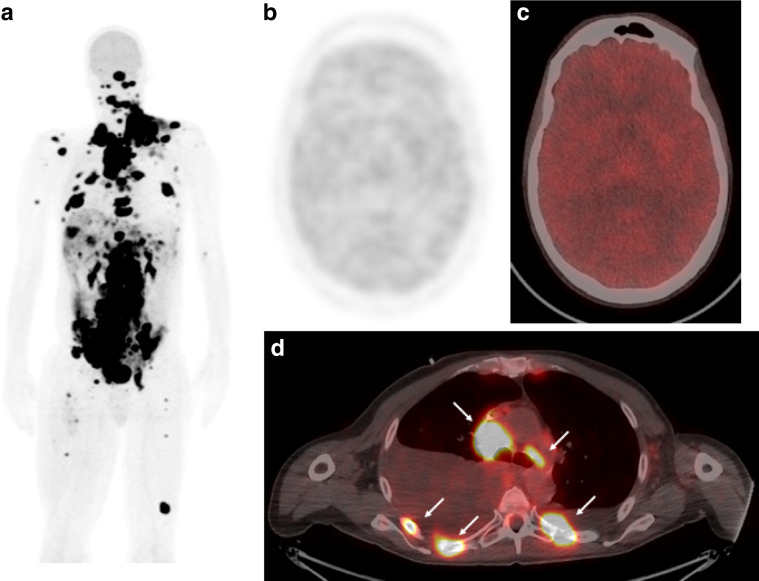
FDG PET showing extensive uptake in tumour metastases throughout the body on maximum intensity projections (a). Reduced uptake in the brain (b, c) is shown on the maximum intensity projection and fused axial images (hot iron scale) and compared with a cross section of FDG-avid nodal and bone metastases in the thorax (d, indicated by white arrows). The PET scan was performed on a GE 710 PET-CT scanner with a dose of 3.5 MBq/kg 18^F^ fludeoxyglucose in 3-min bed positions.
